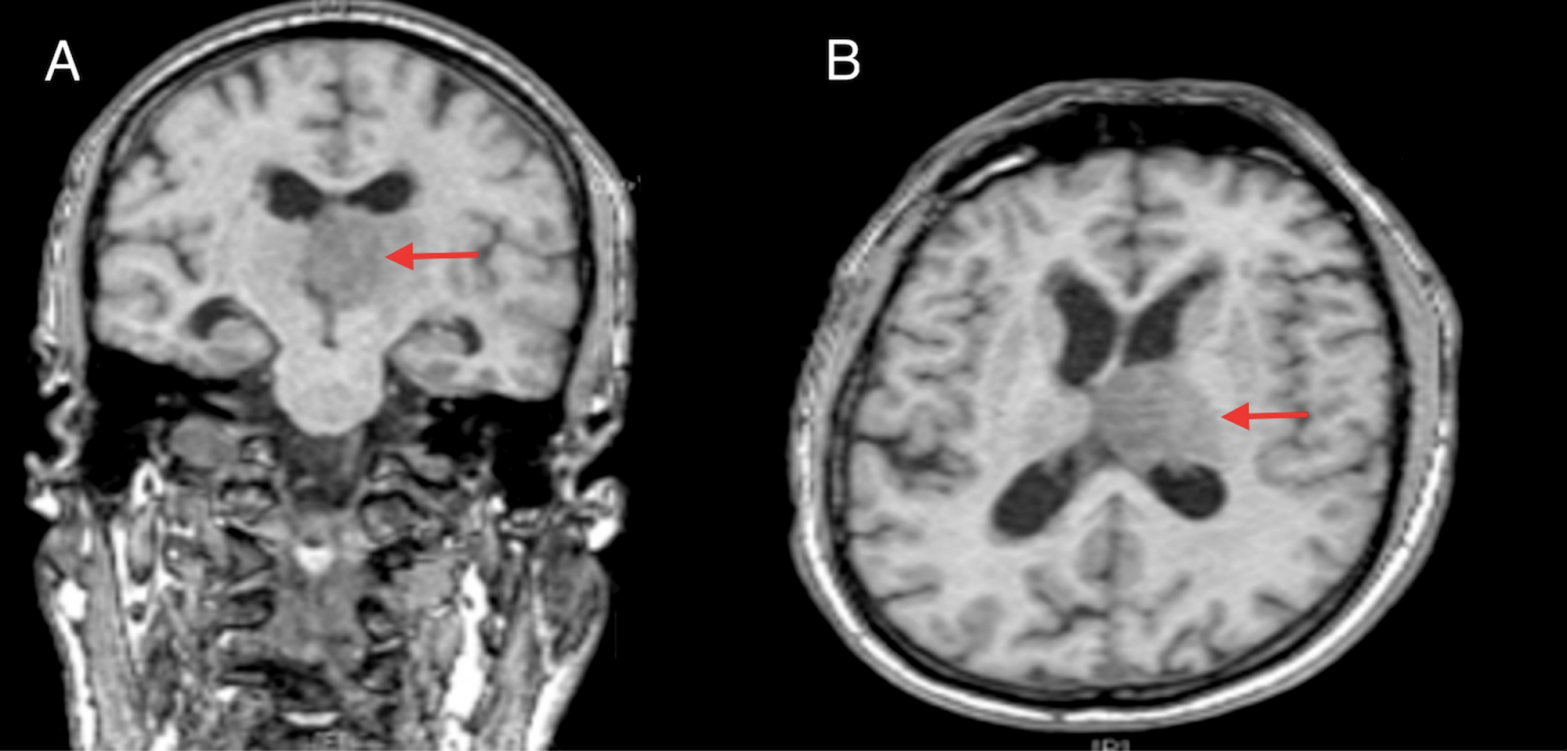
T1-weighted magnetic resonance imaging showing a left thalamic mass (red arrows): (A) coronal and (B) axial views
